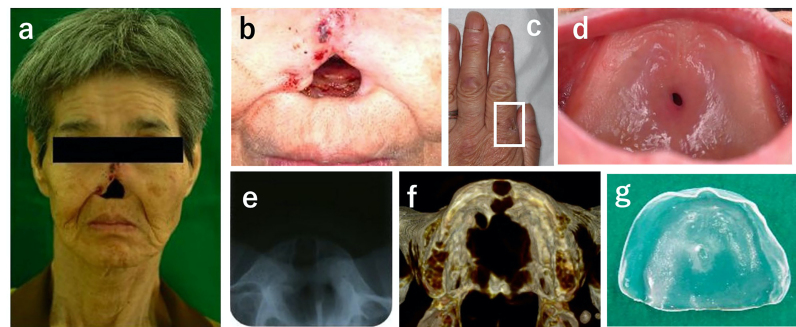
Clinical findings at the initial presentation and the fabricated intraoral appliance (2006 at age 67). (a) Facial photograph; (b) close-up view of the nasal defect; (c) crusted lesion on the dorsal aspect of the left hand (white box); (d) maxillary intraoral photograph; (e) maxillary occlusal radiograph; (f) three-dimensional computed tomography (3D-CT) image; (g) palatal obturator.
